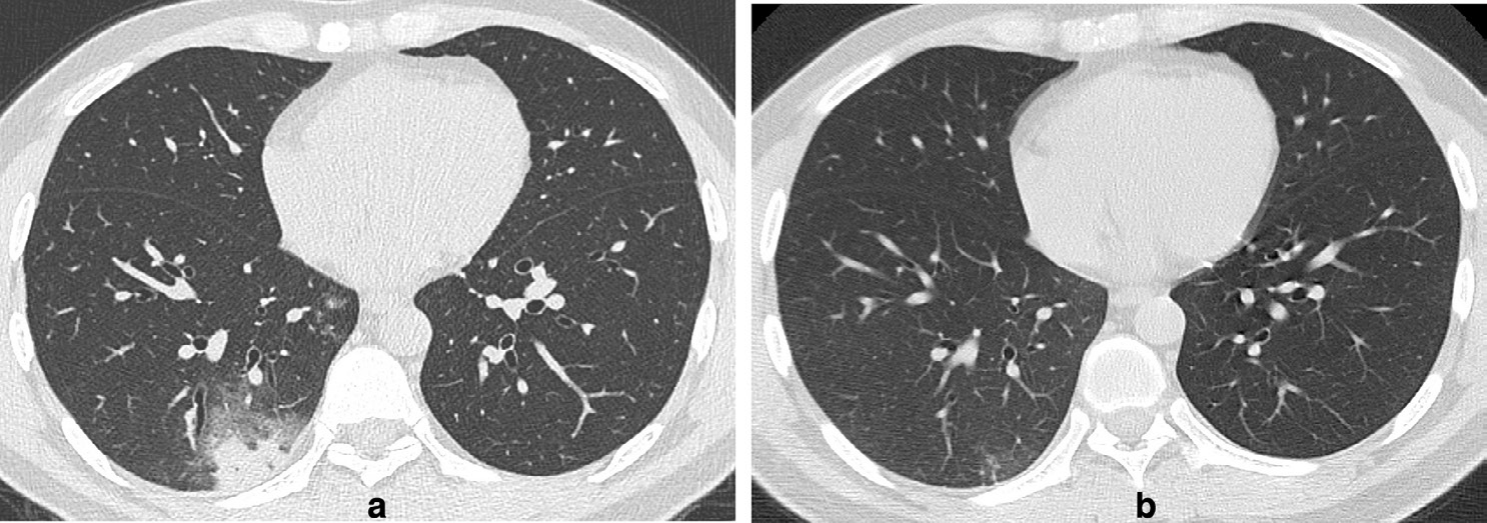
(a-b) A31-year-old male with a history of travelling on a cruise presented with fever, diarrhoea, sore throat and fatigue. (a) Axial thin-section non-contrast CT image on February 1, 2020, showed peripheral/subpleural, patchy, rounded, mixed GGOs in the right lower lobe, with interlobular septal thickening and crazy-paving patterns. (b) Follow-up CT image on February 9, 2020, showed that the lesions were obviously resolved after anti-infection therapy. Nasopharyngeal swabs for SARS-CoV-2 RNA were negative.
